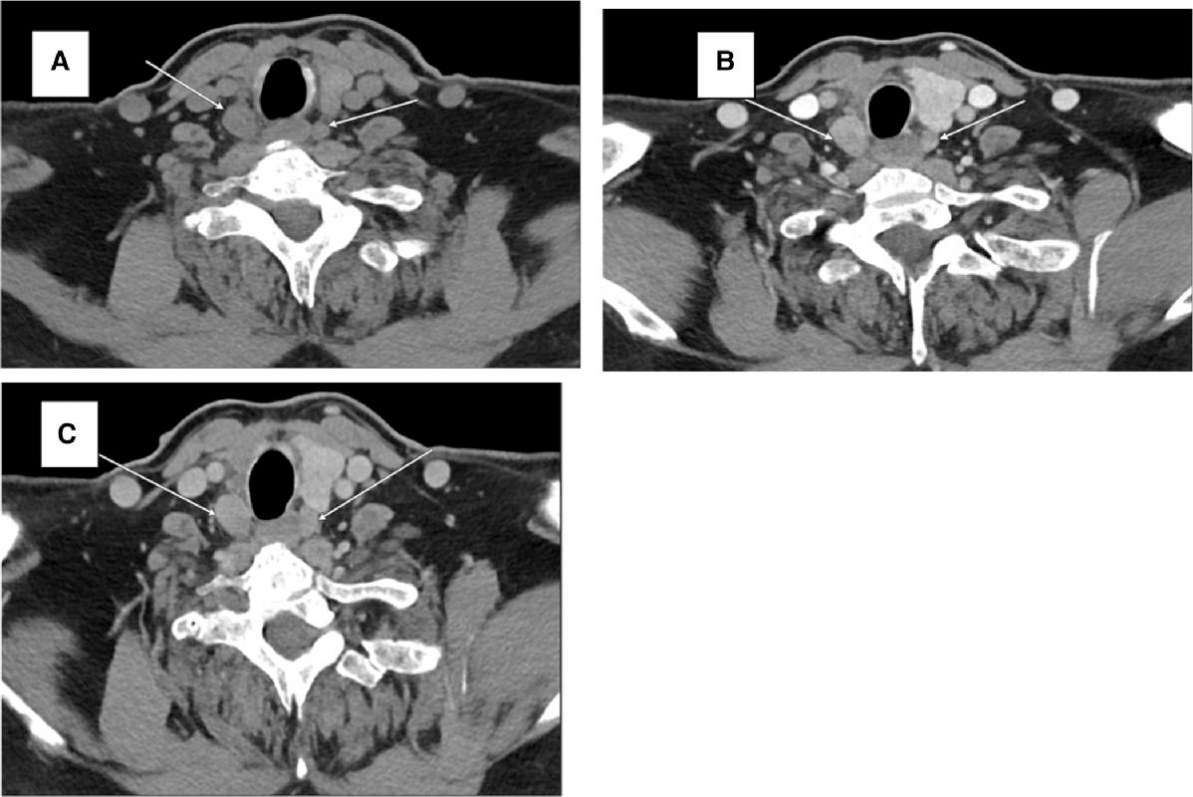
(A) Non-contrast-enhanced axial CT image and (B) IV contrast-enhanced axial CT images during the arterial (20 seconds) and (C) venous (120 seconds) phases of enhancement demonstrated a 1.8 × 1.4 × 3.0 cm and a 1.2 × 0.8 × 2.1 cm (AP × TRV × SAG) hypo-dense lesion with homogenous arterial phase enhancement and delayed phase washout at the level of the cricoid cartridge undersurface, consistent with parathyroid adenomas (white arrows). Additionally, a 0.3 × 0.4 × 0.4 cm parathyroid adenoma was identified posterior to the right carotid artery at the level of the superior aspect of the cricoid cartridge (not shown).
